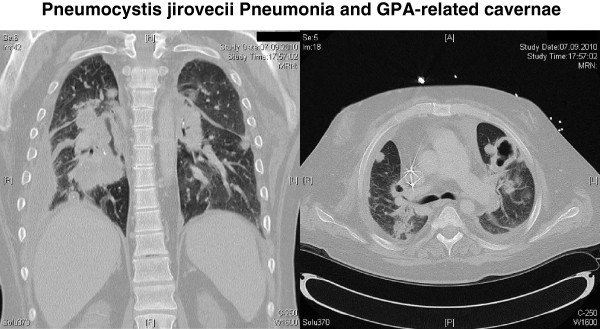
Representative thoracic computed tomography scan at the time of hospitalisation for Pneumocystis jirovecii pneumonia (PCP).
